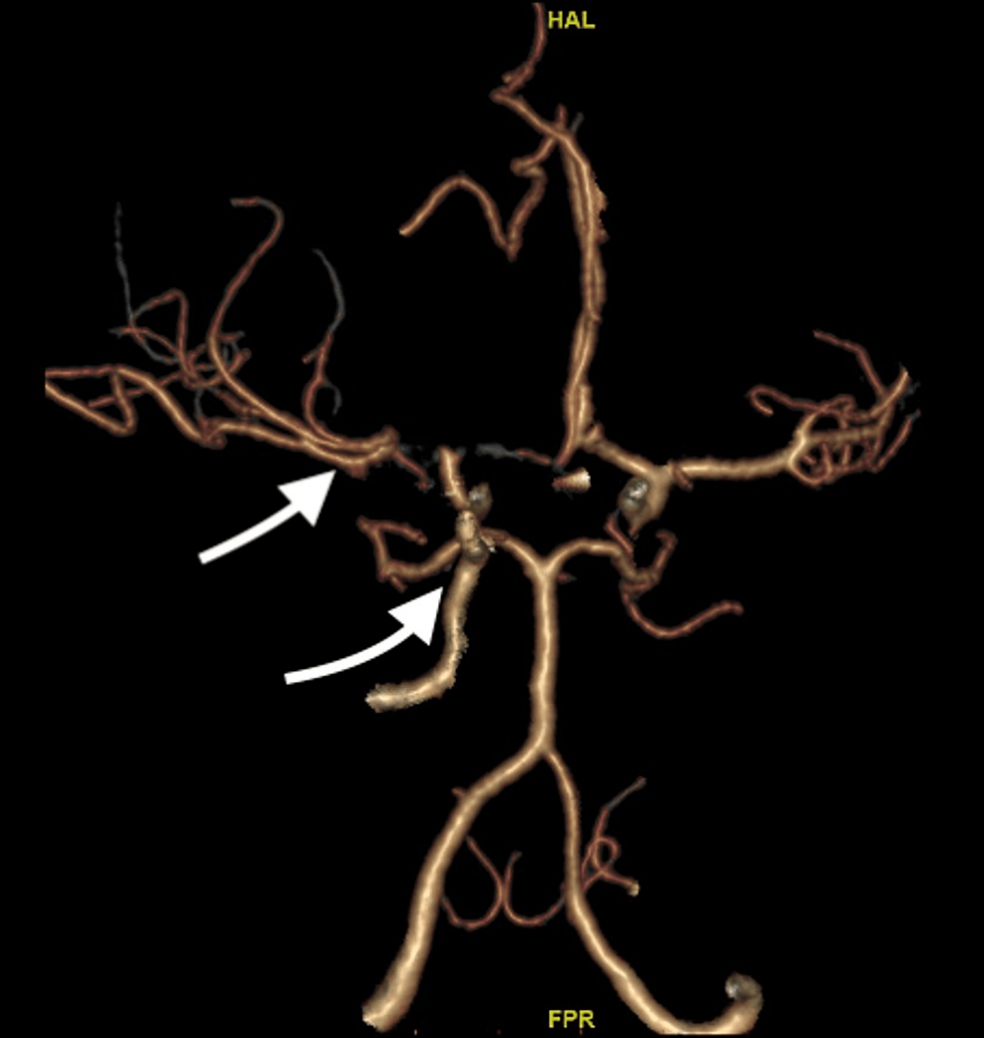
CT angiogram of the head with contrast. Tapered occlusion of the right carotid terminus with non-opacification of the right M1 middle cerebral artery (MCA) (curved arrow). There is distal opacification of the right MCA bifurcation and M2 vessels (straight arrow).
